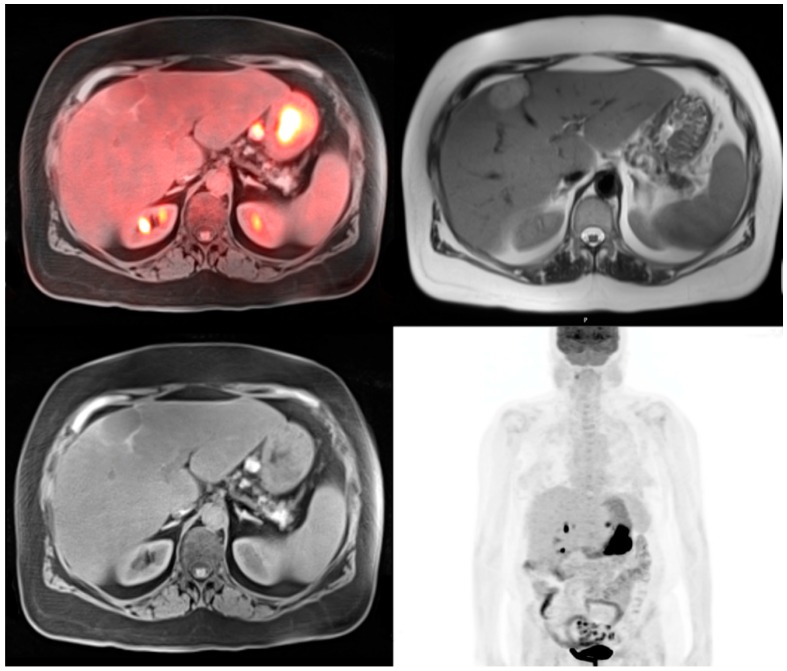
64-year-old female for initial staging of gastric cancer. The PET-MRI transaxial fusion image shows intense hypermetabolic activity in the gastric cancer with SUV 29.2 and a hypermetabolic enlarged gastrohepatic lymph node (*top left*). T1 radial vibe transaxial image with fat suppression shows the gastric cancer to be of low to isointense signal and the metastatic lesion to be hyperintense (*bottom left*). T2 haste transaxial image shows a hyperintense liver lesion that was not active on the PET images which is consistent with a hemangioma (*top right*). The PET MIP image shows the intense activity in the stomach with activity in the adjacent gastrohepatic lymph node (*bottom right*).
